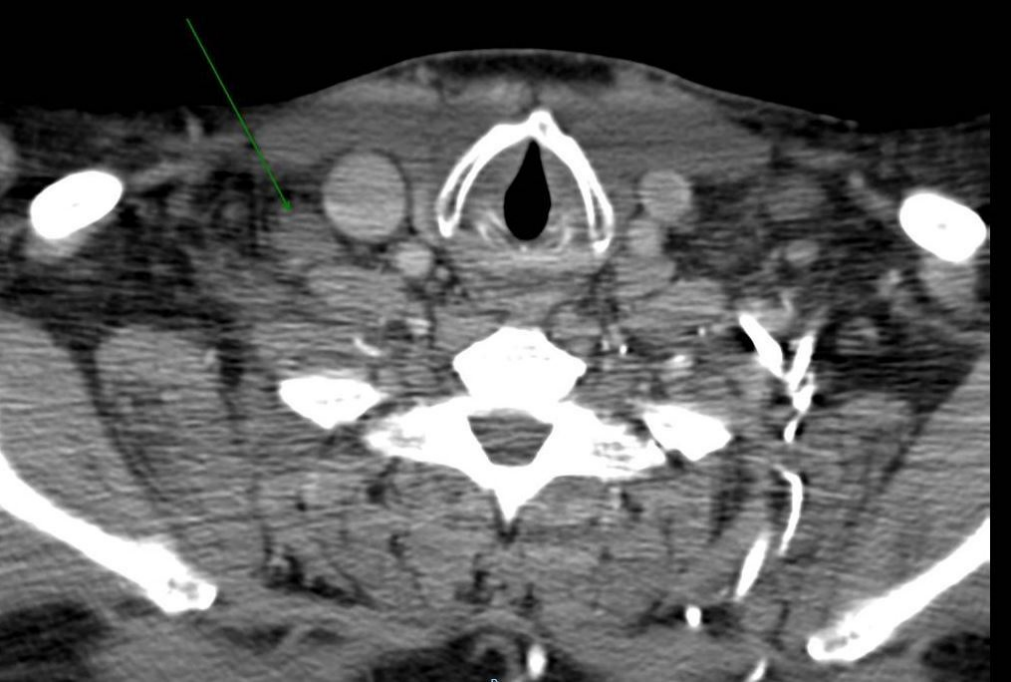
Computed tomography (CT) scan of the chest. The arrow in the scan shows a soft tissue mass that encases and compresses the superior vena cava and right posterior trachea originating from the right lung apex. The tracheal diameter is overall enlarged. Bilateral hilar lymphadenopathy is also evident, but appreciated more on the right side.
